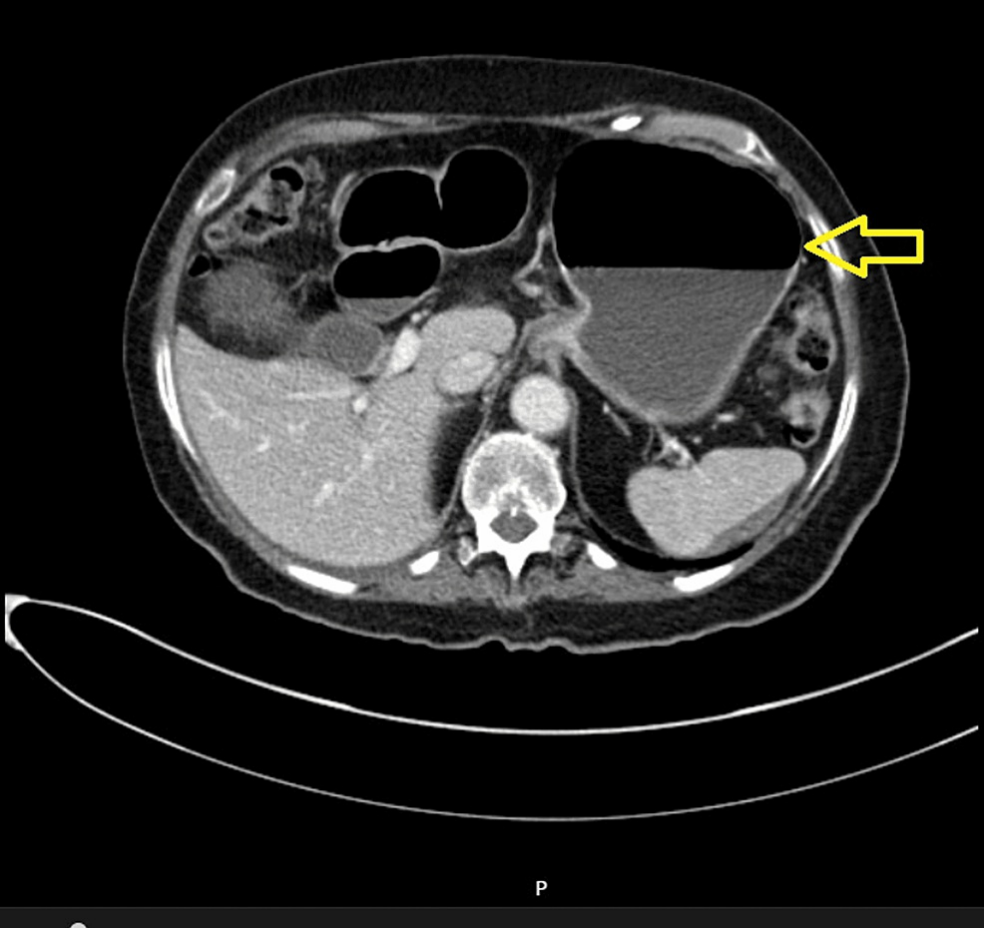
CT scan after gastrografin administration revealed a distended stomach with an air-fluid level inside.
